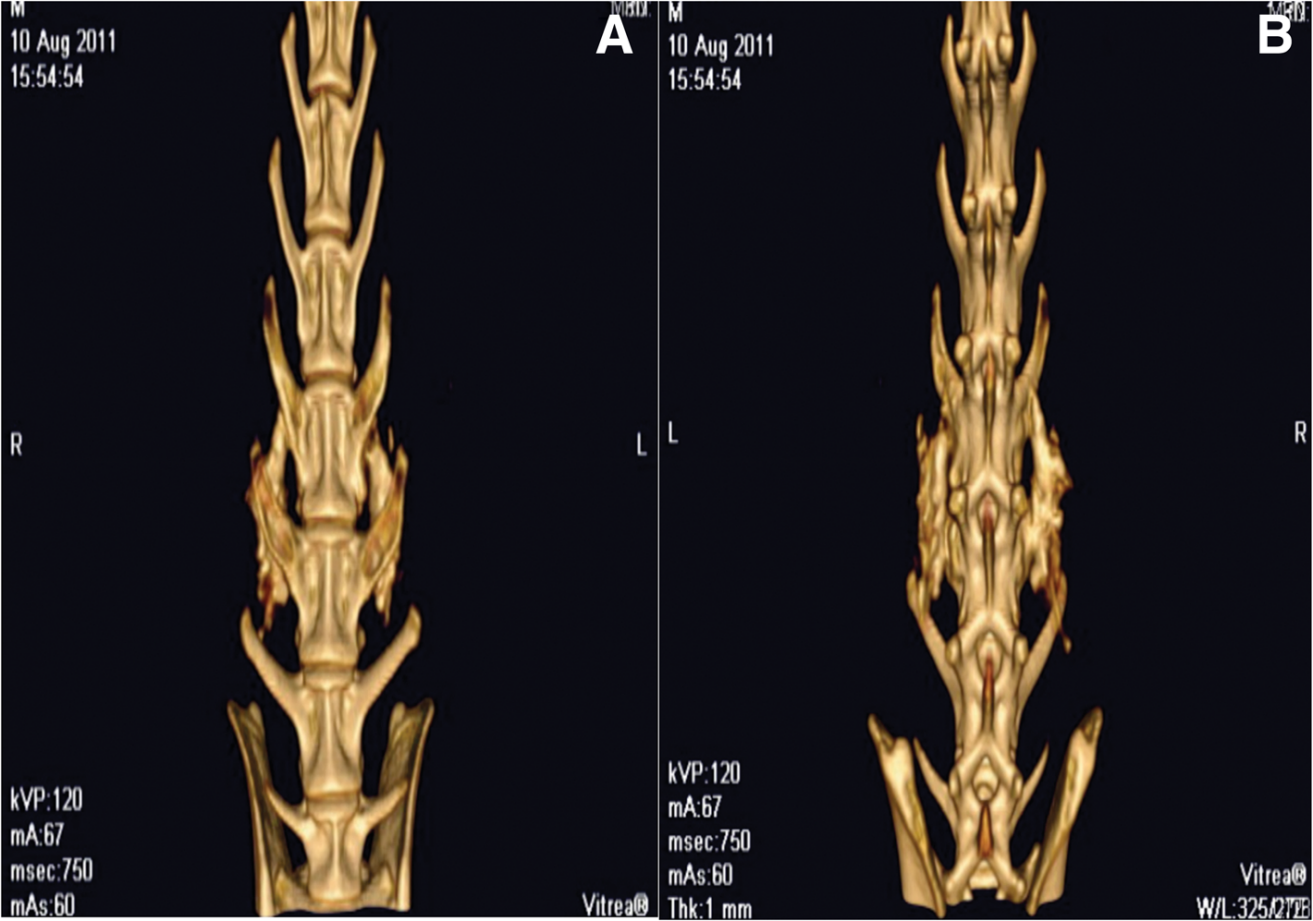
Computed tomography findings. Three-dimensional CT reconstructions revealed the presence of continuous bone bridges and satisfactory fusion mass incorporated with the transverse processes (a, b)
